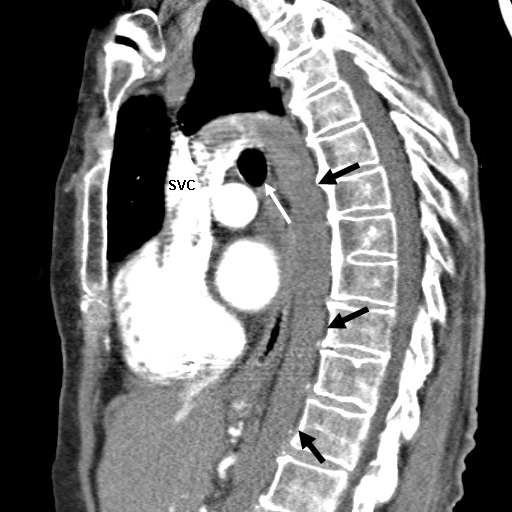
Chest CT with contrast, sagittal section, shows the azygos vein (black arrows)looping over the right bronchus (white arrow) and draining into the SVC just prior to entering the right atrium.
